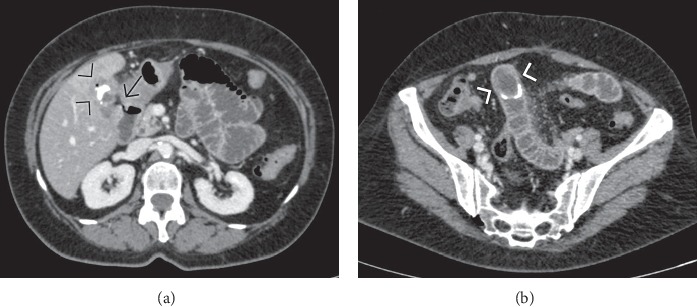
Contrast-enhanced computed tomography in the same patient than Figure 3 allows the depiction of a cholecystoduodenal fistula (arrow) with a linear air collection entrapped in the fistulous communication. Please note in (a) a small air bubble in the gallbladder lumen not detectable at abdominal radiograph, and rim calcification of the gallbladder wall (porcelain gallbladder; arrowheads). Dilated fluid-filled small bowel loops and decompressed distal small bowel consistent with obstruction are evident in (a) and (b). The point of transition corresponds to an isoattenuation intraluminal filling defect with a thin peripheral calcified rim.
